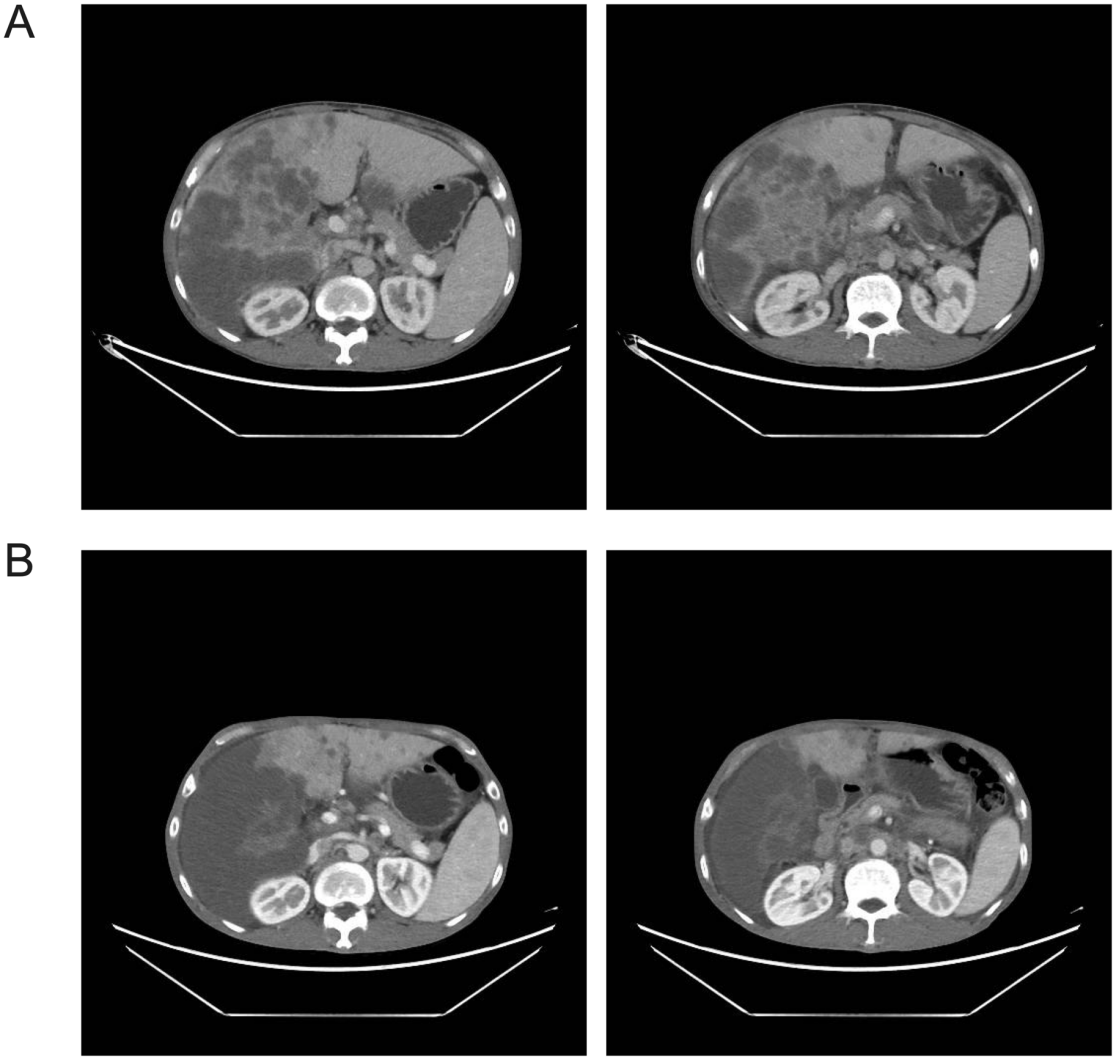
Abdominal CT (A 2024-2-20 vs B 2024-04-22). The liver demonstrates diffuse nodular and mass-like hypodense lesions, with the largest cross-sectional dimension measuring approximately 4.34 cm × 3.49 cm. Some lesions show coalescence with ill-defined margins, predominantly in the right hepatic lobe. Multiple small retroperitoneal lymph nodes are noted (A). The hepatic parenchymal masses and nodules have further coalesced into conglomerates with decreased density compared to prior imaging. New hypodense nodules are noted in the left hepatic lobe with increased number relative to previous examination. No significant change is observed in the ascites within the peritoneal and pelvic cavities (B).
